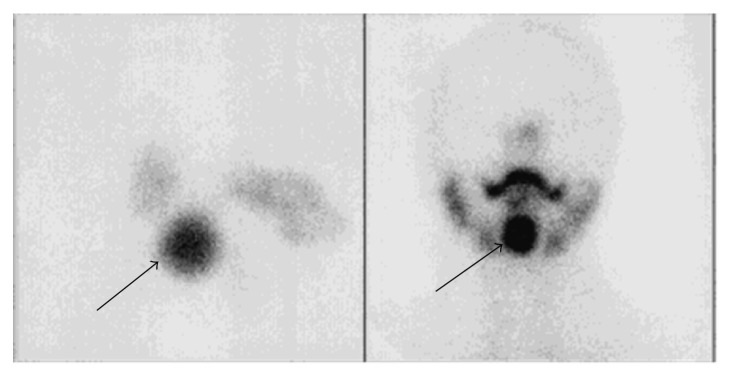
Thyroid scintigraphy: intense and elective uptake at the base of the tongue.
